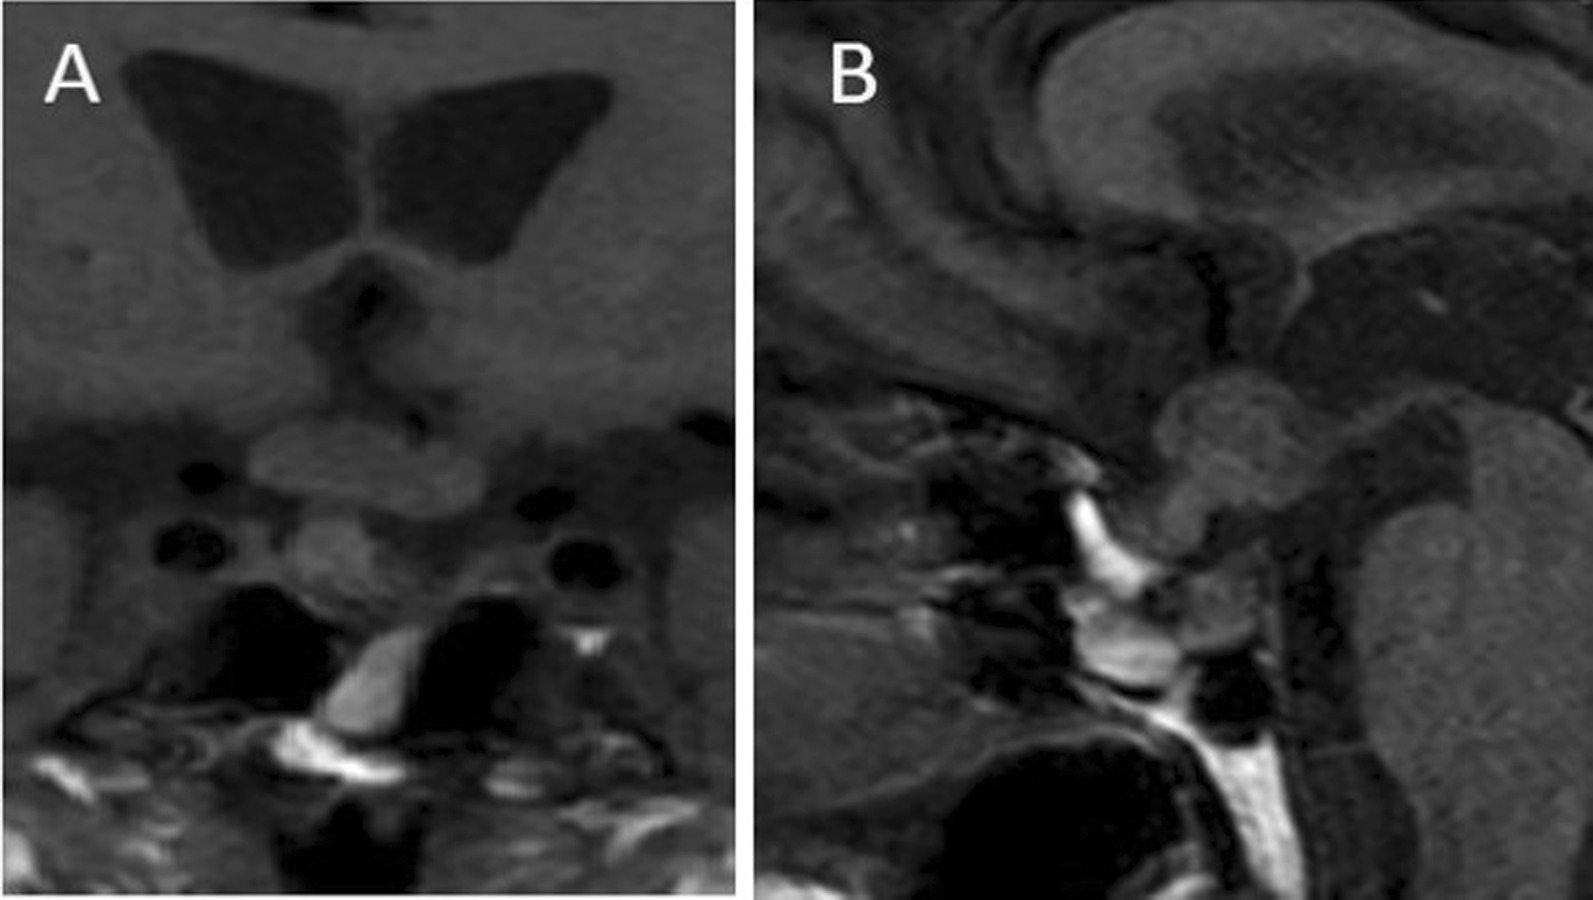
Focused sellar MRI study confirming the presence of a mass, isointense with the surrounding brain parenchyma in T1-weighted sequences and extending from the anterior pituitary towards the peduncle and the optical chiasma (the brain CT performed at emergency room (ER) admission demonstrated a sellar mass of about 17 × 12 mm), in coronal (A) and lateral (B) projection
